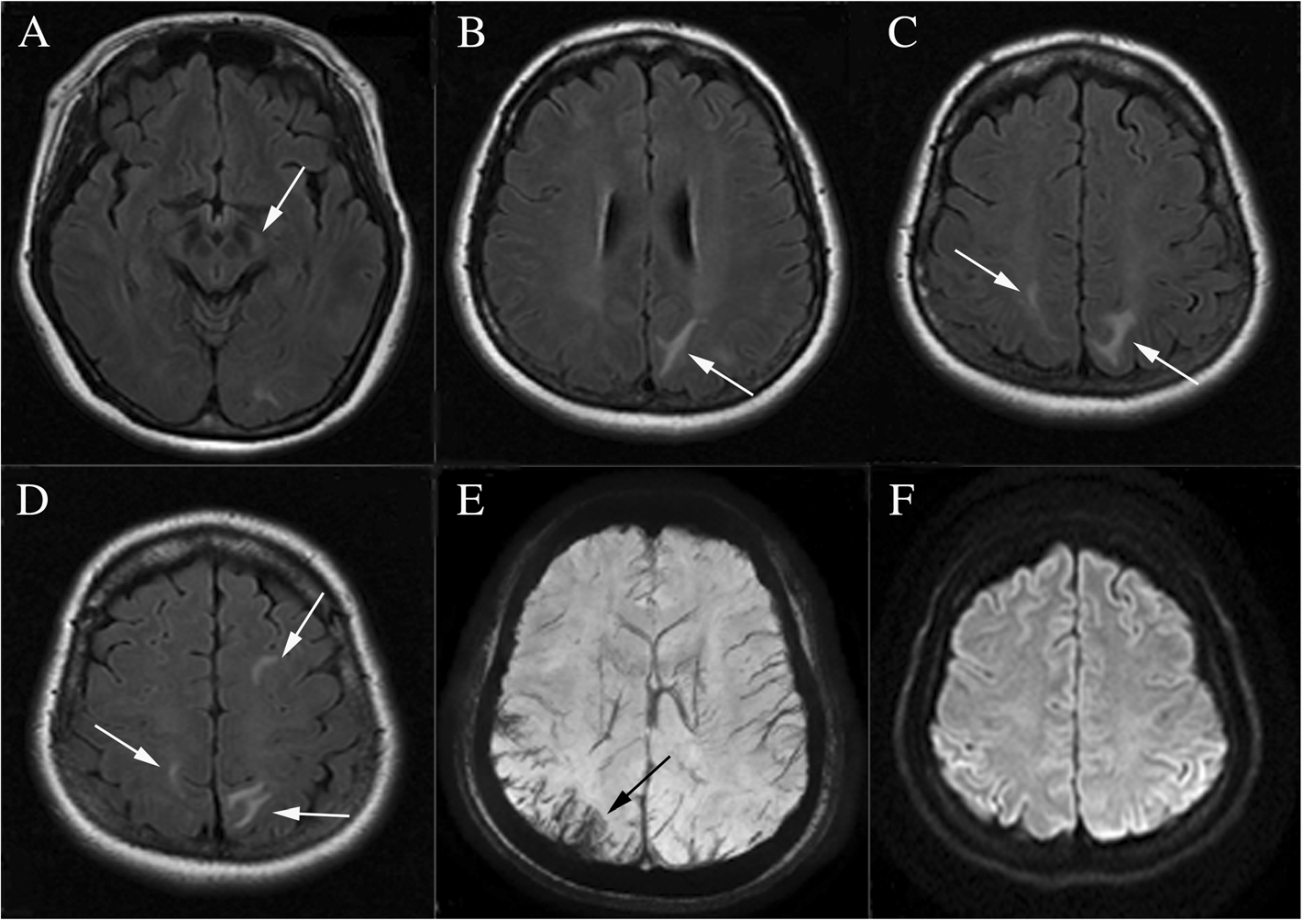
Axial FLAIR (a-d) demonstrates PRES lesions involving multiple regions (white arrows). SWI (e) reveals low signals in the right parietal-occipital sulci. There are no abnormal signals in DWI (f)
